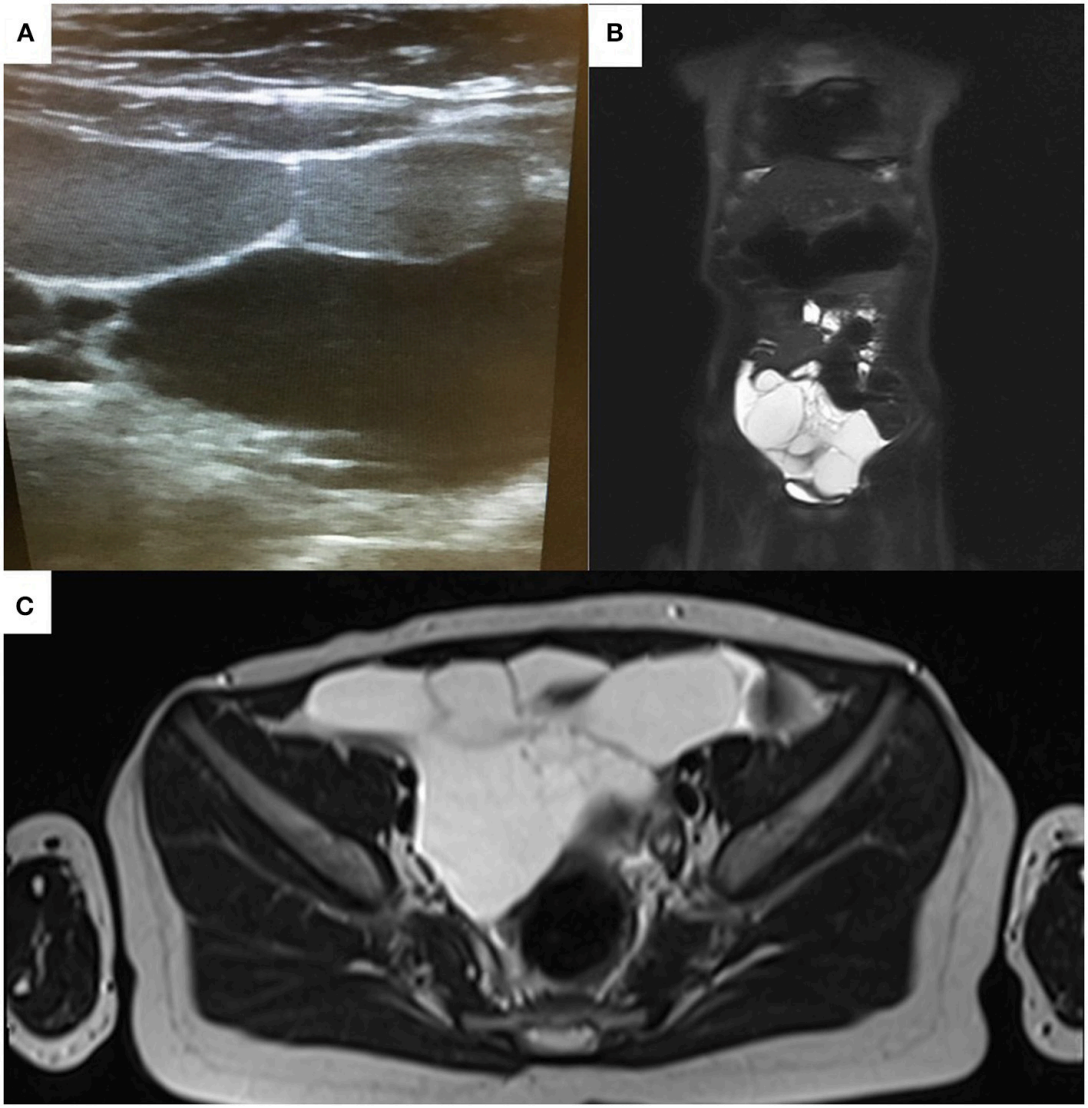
Echographic (A) and MRI appearance (B,C) of the abdominal tumor mass initially misdiagnosed as acute appendicitis. Note the multicystic appearance (A).
